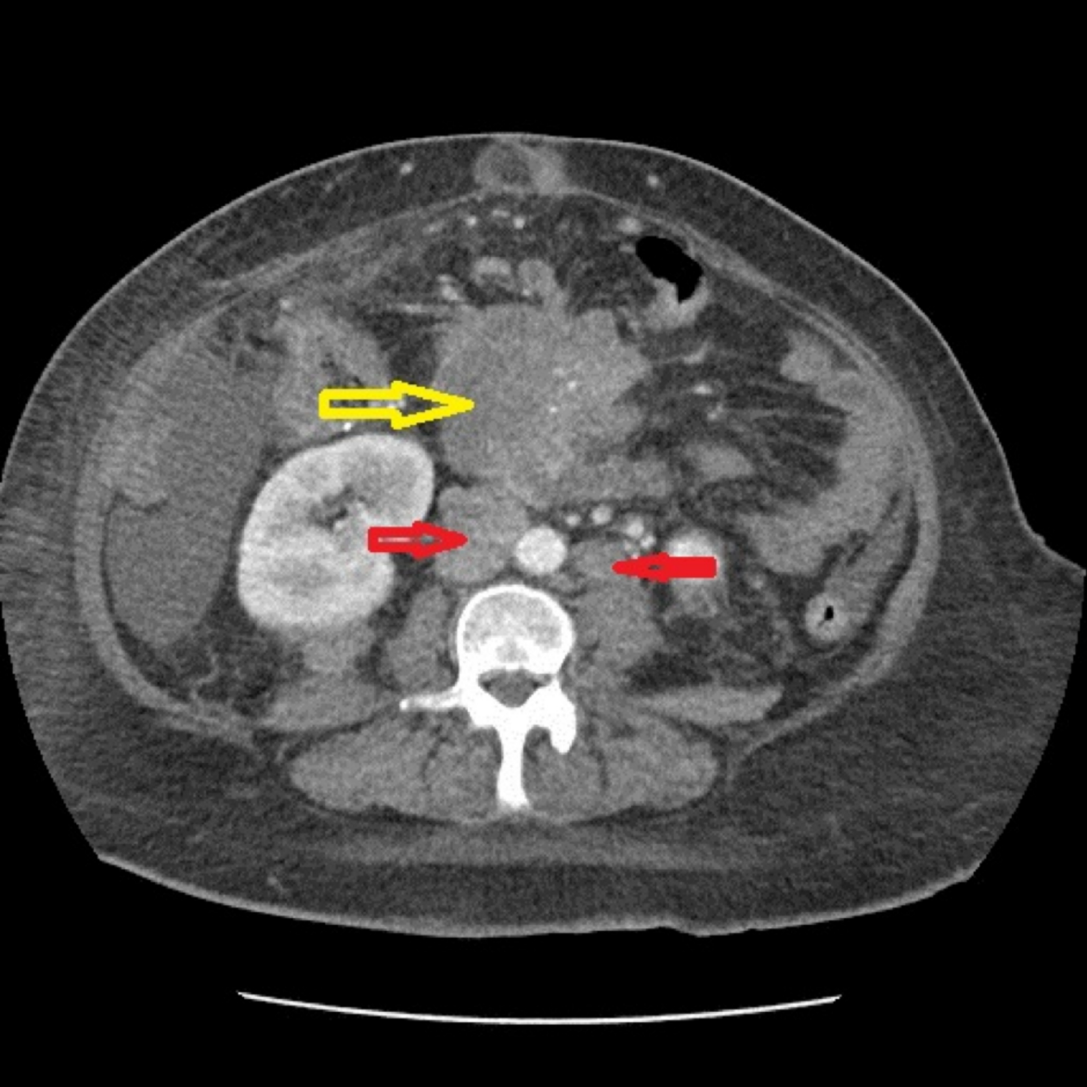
Repeat CT of the abdomen and pelvis in August 2018 - coronal view 2 The image shows an enlarged mesenteric mass (yellow arrow) and retroperitoneal adenopathy (red arrow) CT: computed tomography
